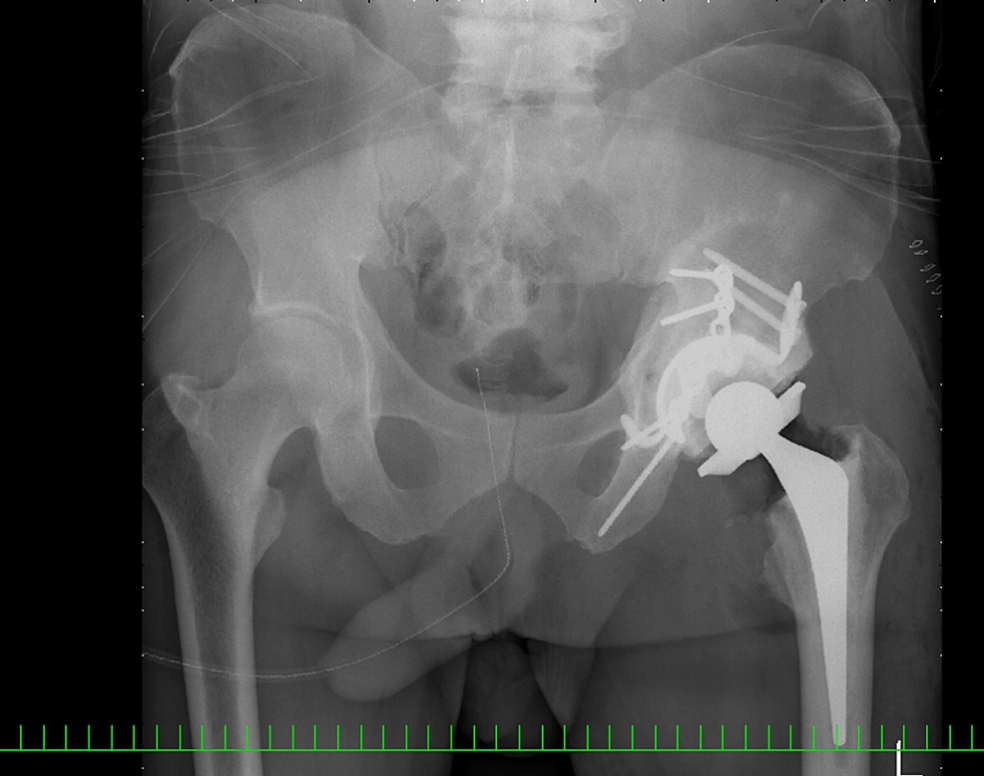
Plain radiograph after the first revision surgery
